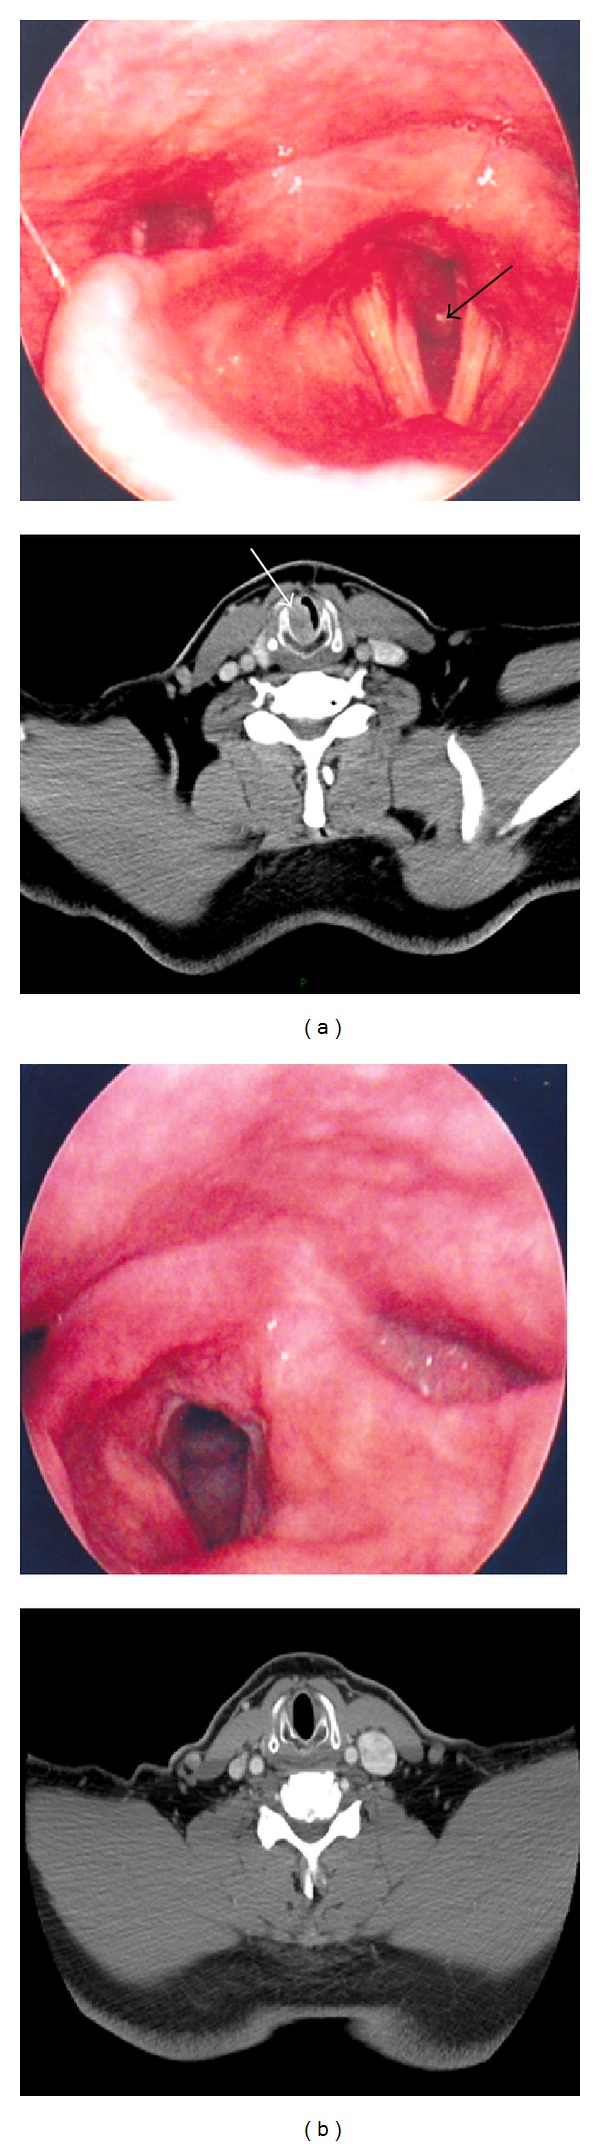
(a) At the top there is an image obtained by indirect laryngoscopy (IL) revealing severe edema of the posterior commissure and a polypoid mass on the right posterolateral subglottic wall (arrow). At the bottom there is an axial image of computer tomography (CT) at the cricoid cartilage level that shows a homogenous image with contrast enhancement at the subglottic submucosal region (arrow). (b) At the top there is an image obtained by IL 6 months after the treatment was finished. It shows absence of the subglottic mass. At the bottom there is an axial CT image at the cricoid cartilage level that reveals 100% free subglottic airway.
